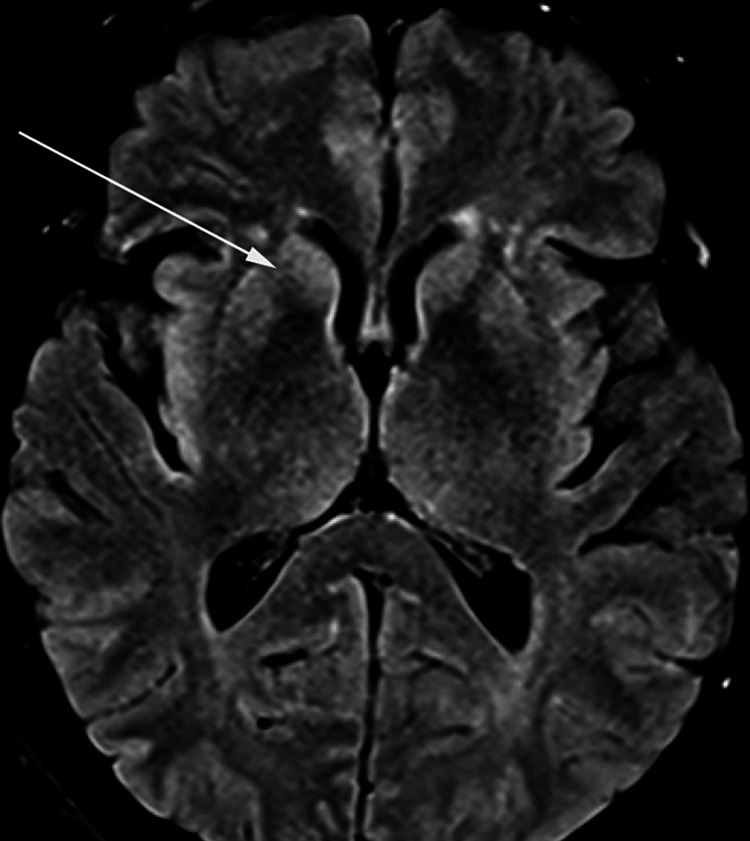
MRI brain showing hyperintensity in the caudate nucleus
